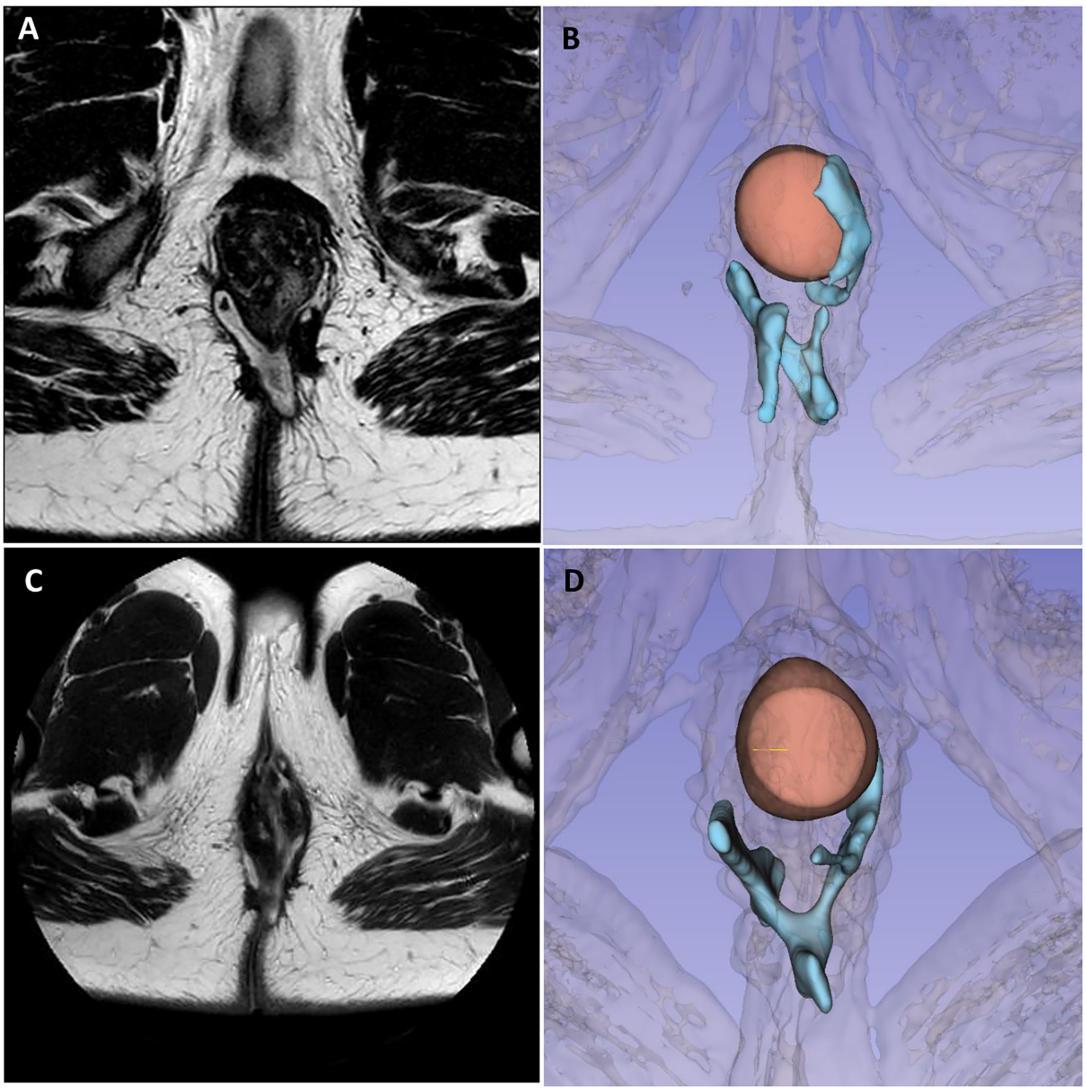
Case 1 imaging pre- and post-surgery. Panels (A, B) show preoperative pelvic magnetic resonance imaging and 3D reconstruction highlighting a complex branched perianal fistulising disease with trans-sphincteric fistulas joining in the posterior plane with two external orifices on the right and one on the left, with a 2-cm abscess in the left ischioanal fat. Panels (C, D) show 6-month MRI follow-up, with the persistence of fistula tracts, less signs of inflammation, less thickening, contrast enhancement, and no fluid collections.
